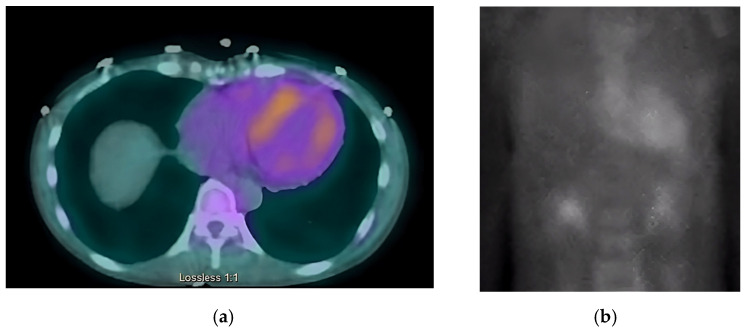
SPECT in an ATTR cardiac amyloidosis patient. Evidence of uptake of ^99m^Tc-PYP in the myocardium. (a) Quantitative SPECT/CT; (b) Semiquantitative analysis of ^99m^Tc-PYP myocardial uptake.
